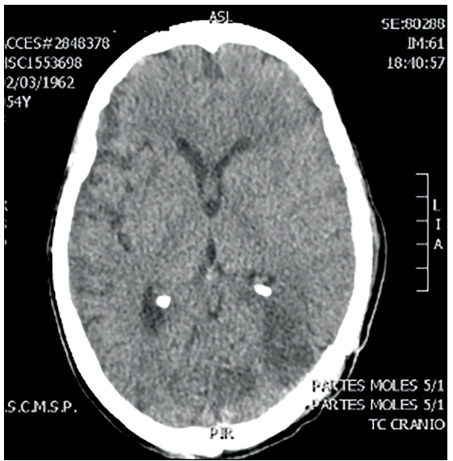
Axial computed tomography performed during the postoperative period showing gross total resection.
